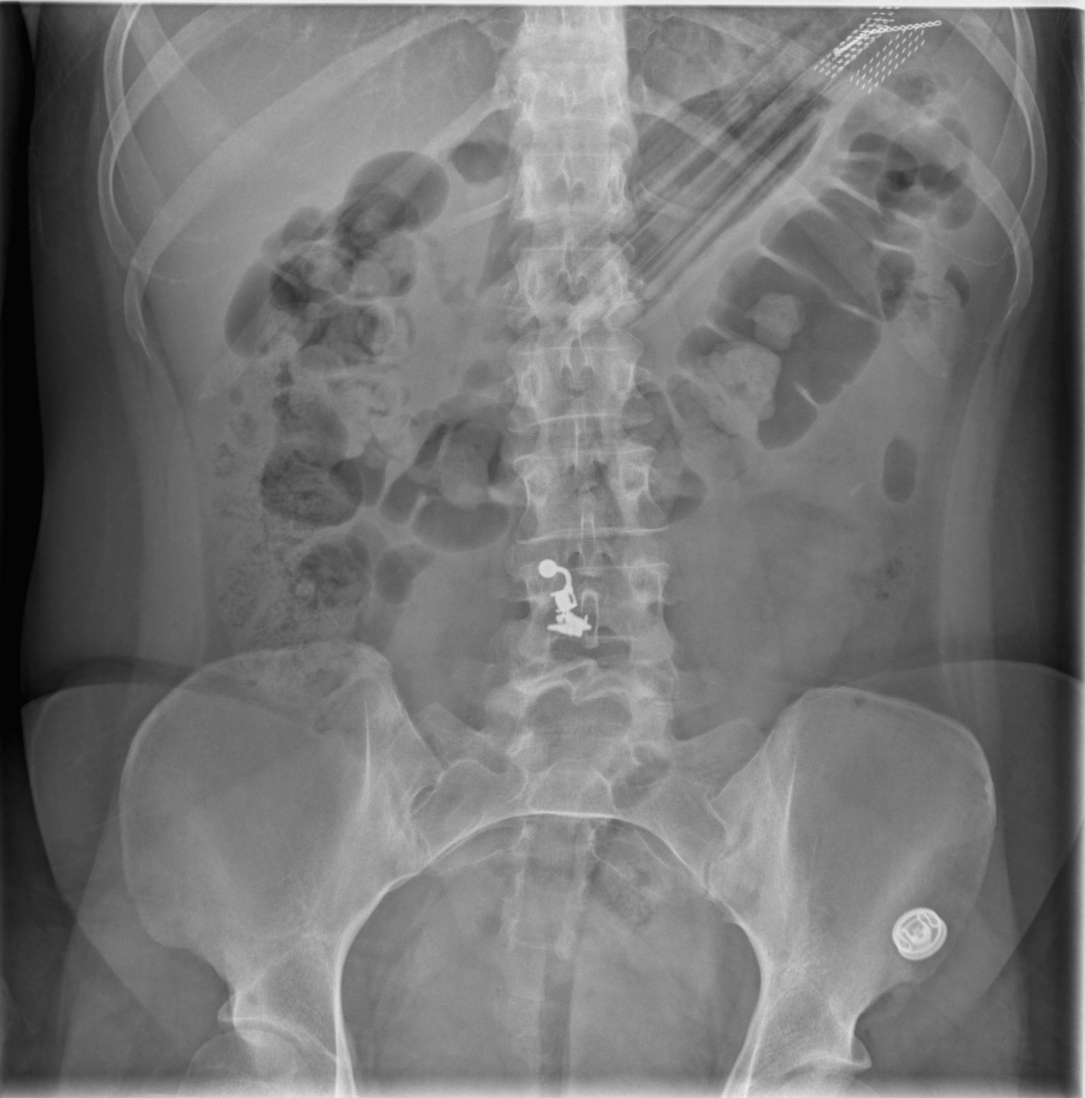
Abdominal X-ray confirming the presence of two toothbrushes in the left upper quadrant identified by their bristles.
